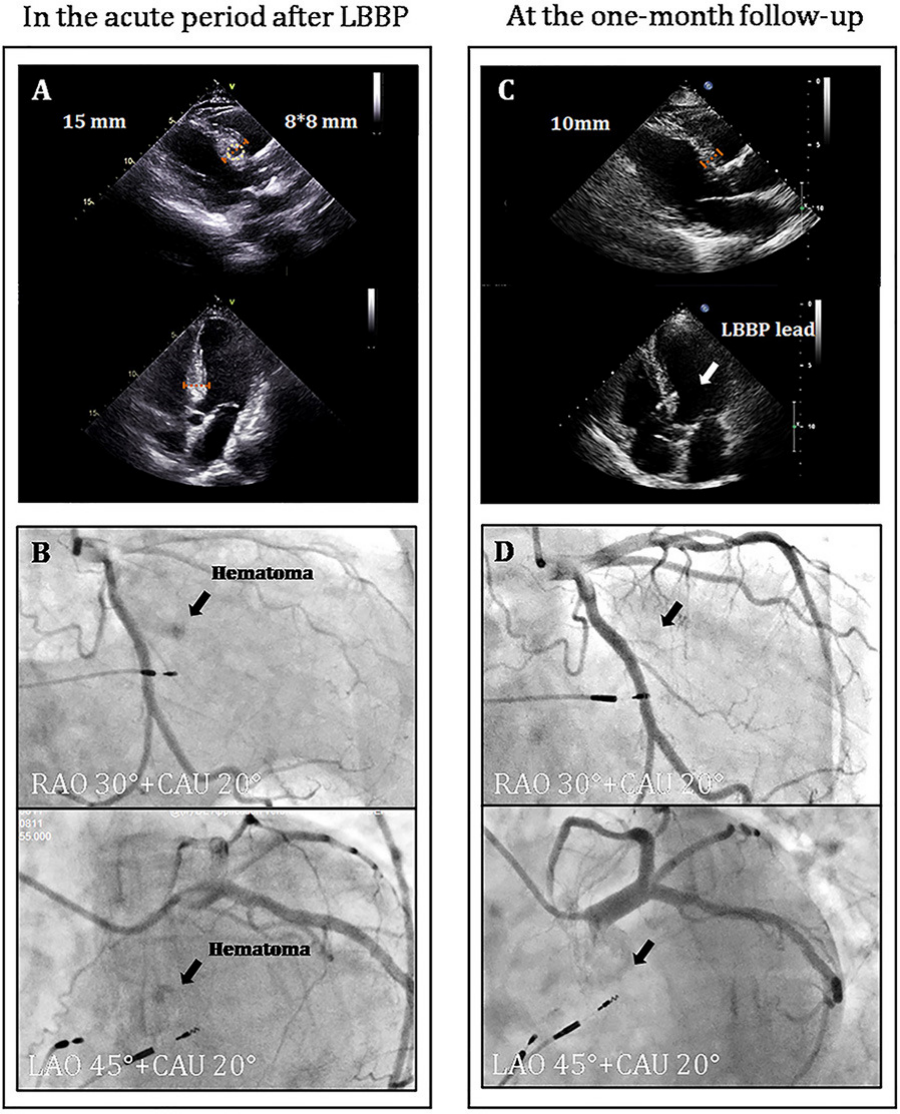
(A) The echocardiogram indicated interventricular septal hematoma measuring 8 mm in width. (B) Contrast agent retention in the interventricular septum. (C) The echocardiography confirmed the hematoma has resolved. (D) No contrast agent overflow was found in the interventricular septum. RAO, right anterior oblique; CAU, caudal; LAO, left anterior oblique.
